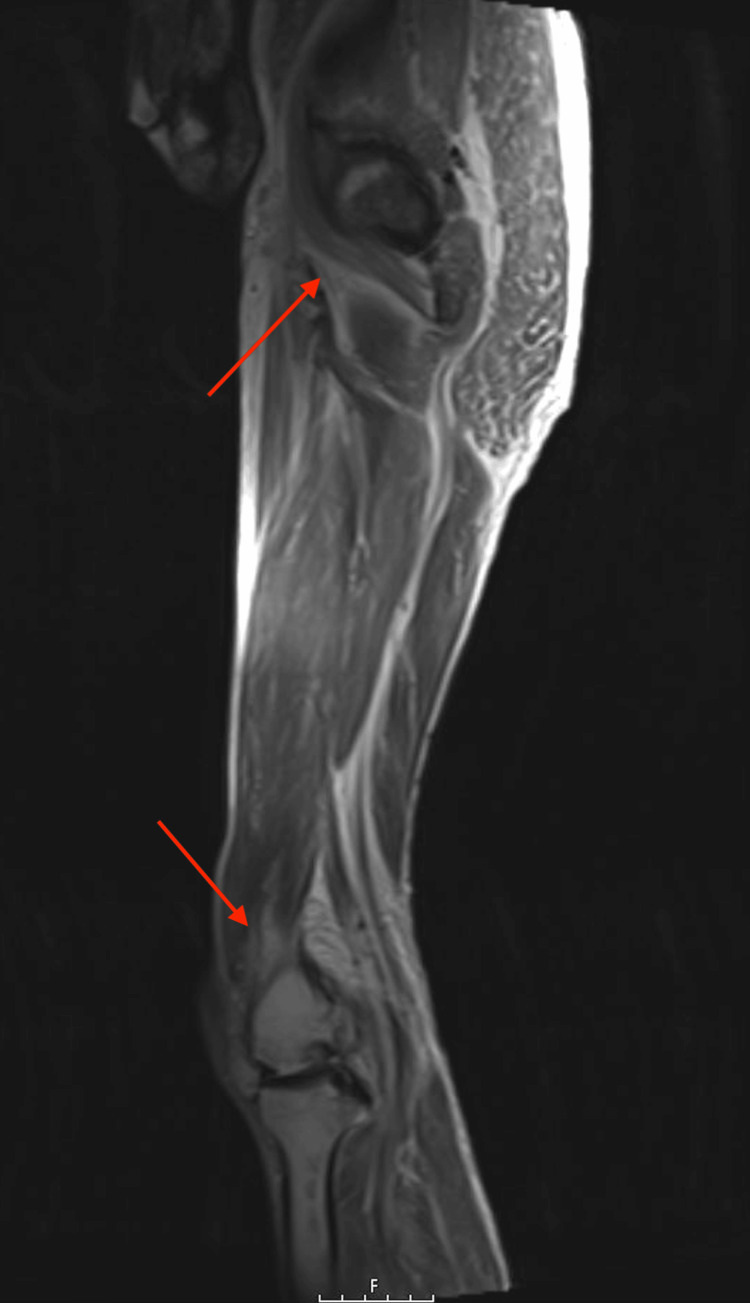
MRI left lower extremity non-joint without contrast showing decreased T1 and increased T2 signal within the subcutaneous adipose tissue (red arrow). MRI: magnetic resonance imaging.
